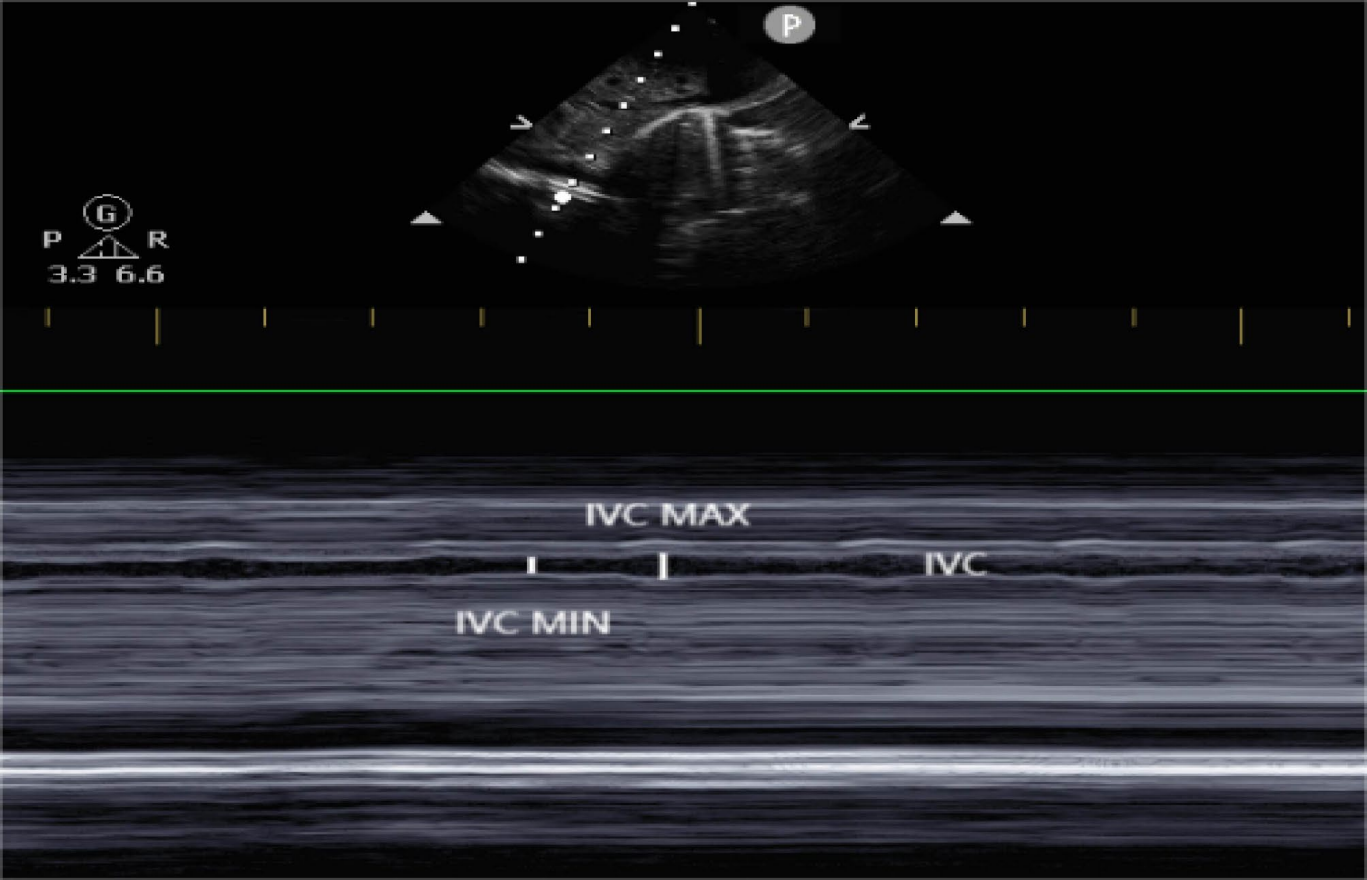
2-D ultrasound showing IVCmin and IVCmax
